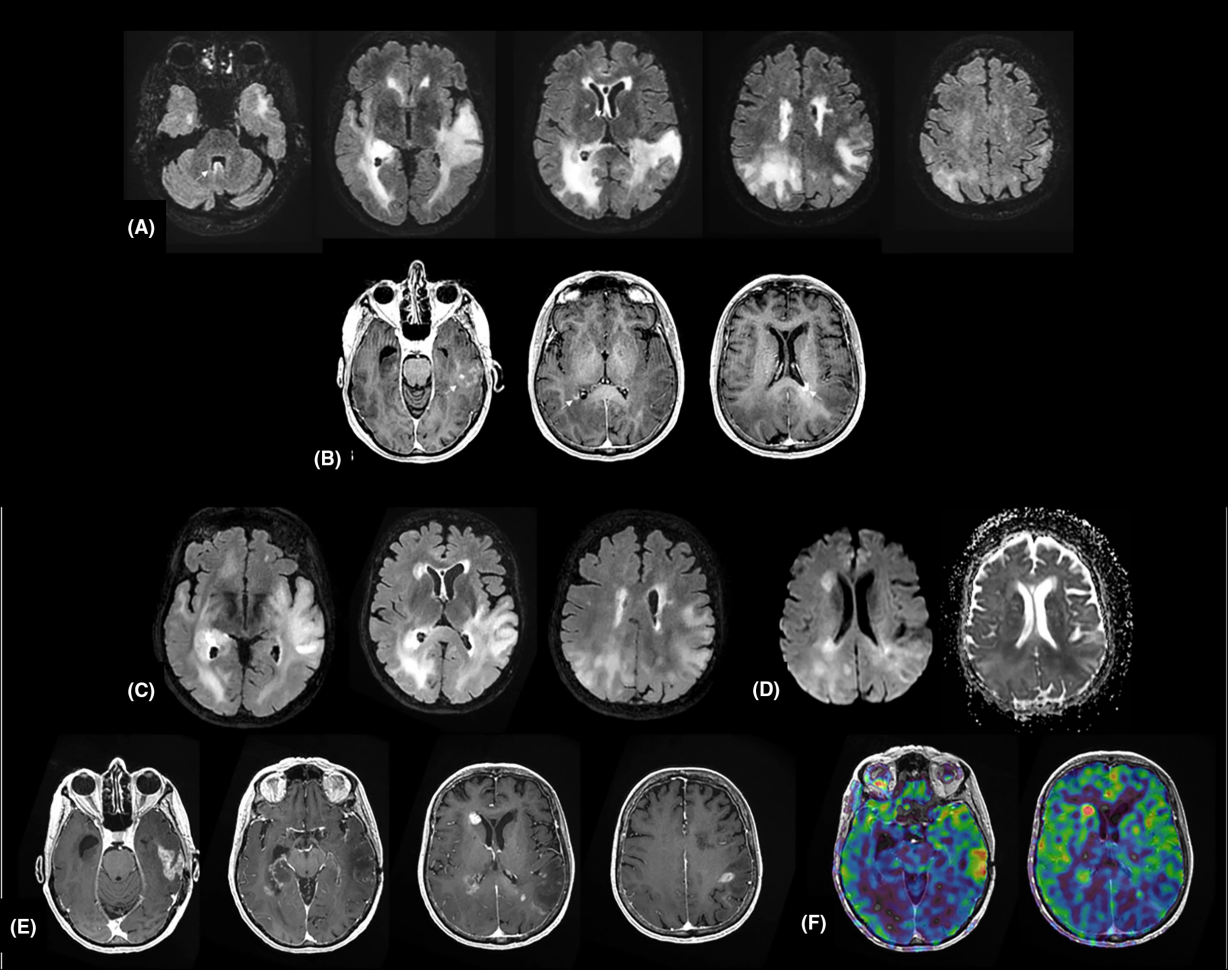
Radiological features. Preoperative brain MRI: (A) 3D FLAIR axial sequence – Diffuse white matter infiltration with predominant involvement of bilateral temporo‐parieto‐occipital regions, with cortical and ependymal extension (arrow). (B) Axial 3D T1‐weighted post‐contrast sequence: Foci of patchy enhancement, cortico‐subcortical left temporal and periventricular (arrow). Follow‐up imaging at 3 months postoperative under Temozolomide treatment: Increased tumour infiltration in T2 FLAIR imaging (C) with hypercellular foci showing hyperintensity on diffusion‐weighted imaging with decreased apparent diffusion coefficient (ADC) on mapping (D). Increased and appearance of enhancing foci (E), some of which are hypervascular, on ASL perfusion imaging (F).
